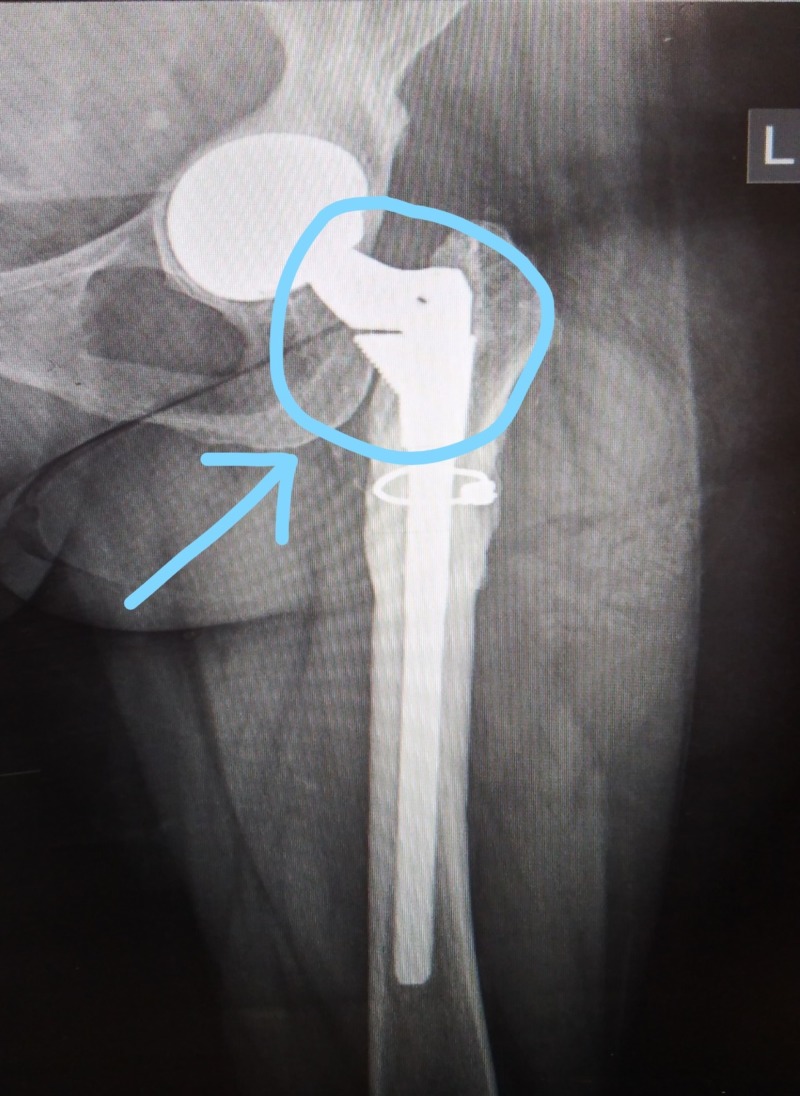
Post-operative X-ray with the 'happy elephant sign'
